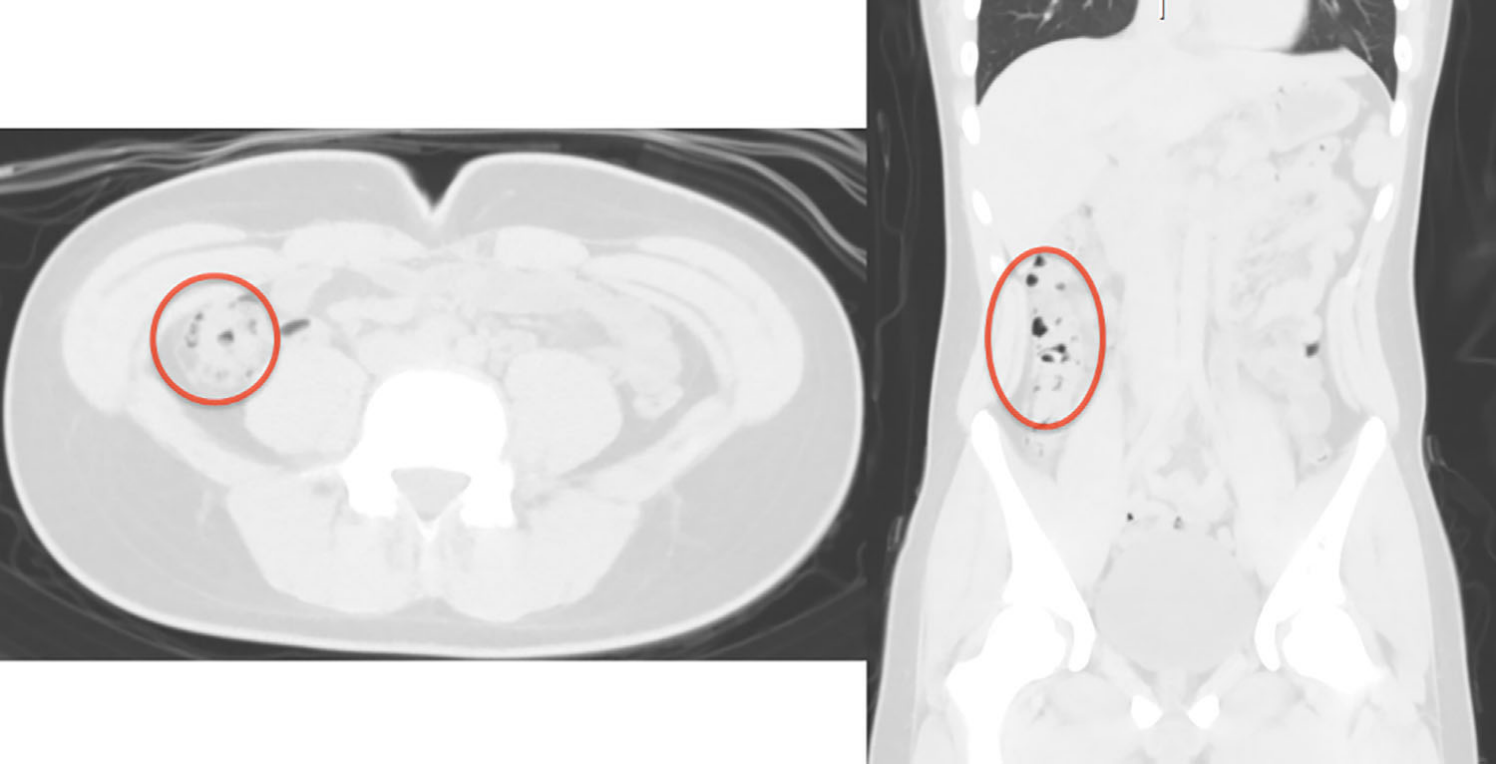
Computed tomography image on day 10 of reconstruction showing the emphysematous lesions had shrunk significantly (red circle).
